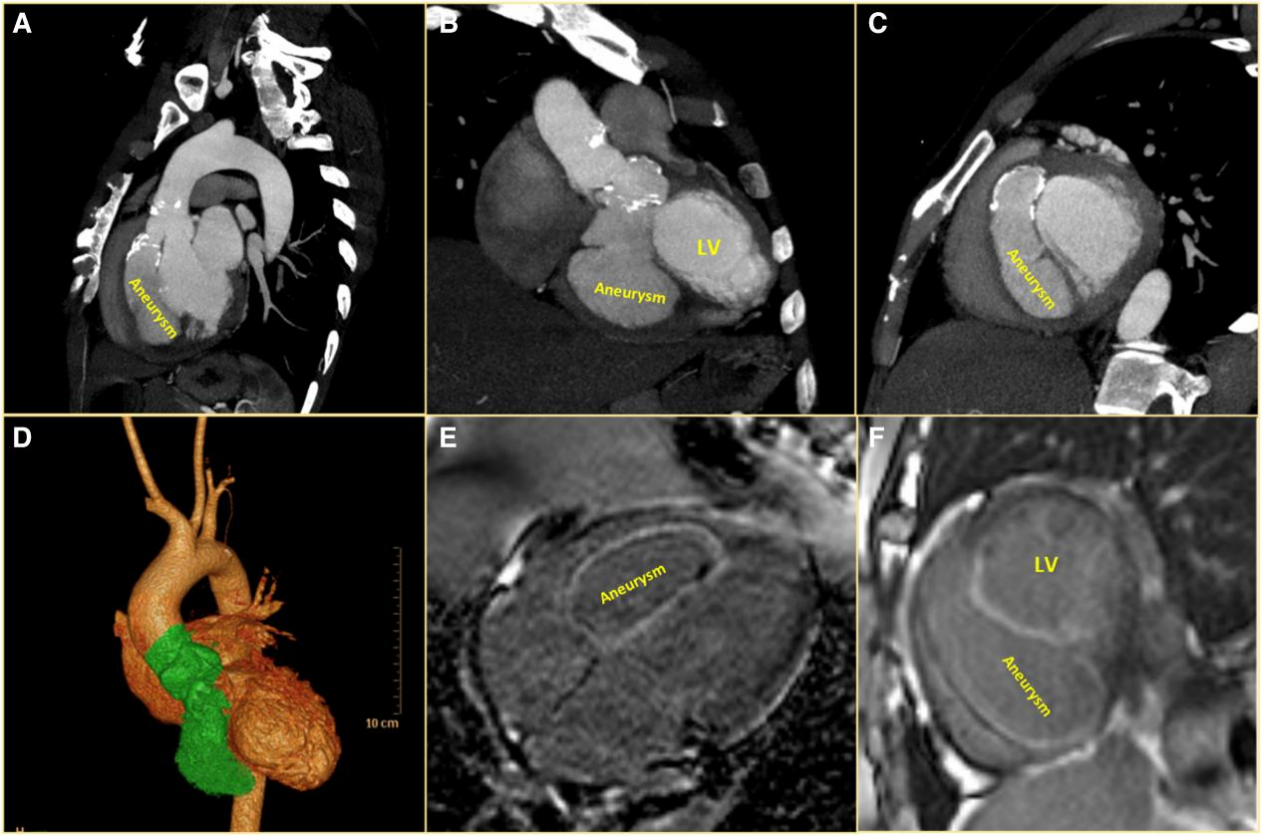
Sagittal (*A*) and coronal (*B*) cardiac computed tomography (CT) images showing the aneurysm arising from the right sinus of Valsalva dissecting into the interventricular septum. Short axis reconstructed CT image (*C*) showing the ‘triple ventricle’ appearance formed by the large dissecting aneurysm. (*D*) CT volume rendered image highlighting the sinus of Valsalva aneurysm in green (shaded in grayscale version). Horizontal long axis (*E*) and short axis (*F*) phase-sensitive inversion recovery MRI images showing the same aneurysm. LV, left ventricle.
